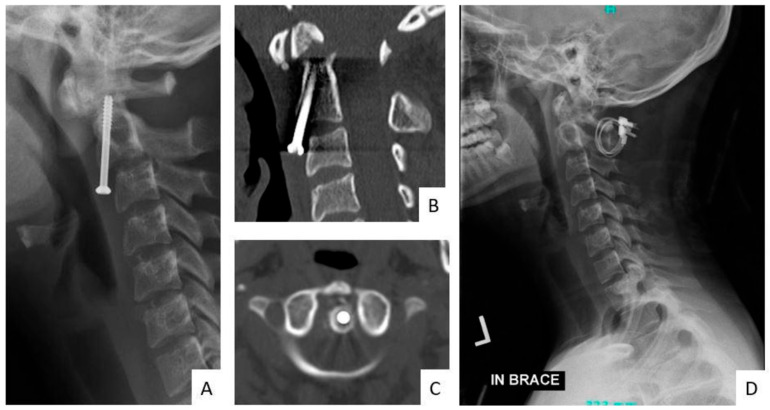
Representative case of delayed implant loosening and revision surgery. Case of an 18-year-old female with a C2 odontoid fracture was treated with odontoid screw fixation. Final intraoperative fluoroscopic images demonstrated acceptable alignment and implant positioning at the index procedure. At approximately 12 weeks postoperatively, follow-up radiographs demonstrated signs of hardware loosening/nonunion (A). CT was subsequently obtained for further characterization (B,C), and the patient ultimately underwent posterior C1–C2 fusion revision surgery (D).
